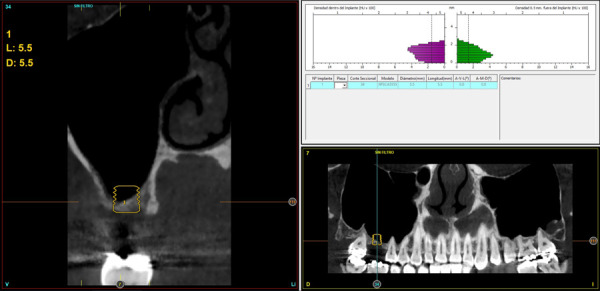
Imagen de planificación del *cone-beam* con el implante de 5,5 mm de altura y 5,5 mm de anchura. Podemos observar como con esta anchura podemos lograr un correcto anclaje vestíbulo-palatino. En el área vestibular, se realizará una pequeña elevación de seno transalveolar para ganar el volumen óseo necesario.
